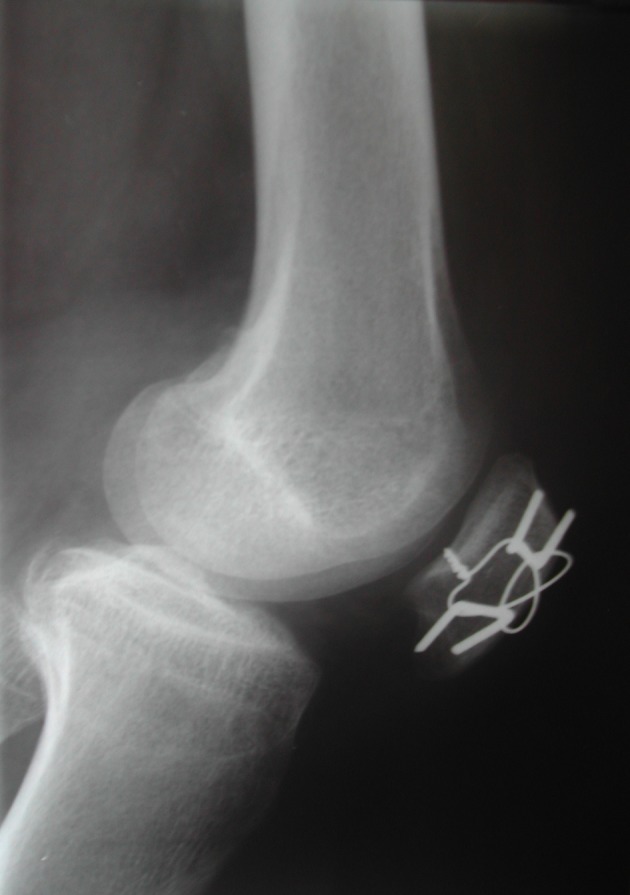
Seven days postoperatively, lateral radiograph demonstrated the total reduction of the patella fracture without joint gap and the use of the modified tension band.
